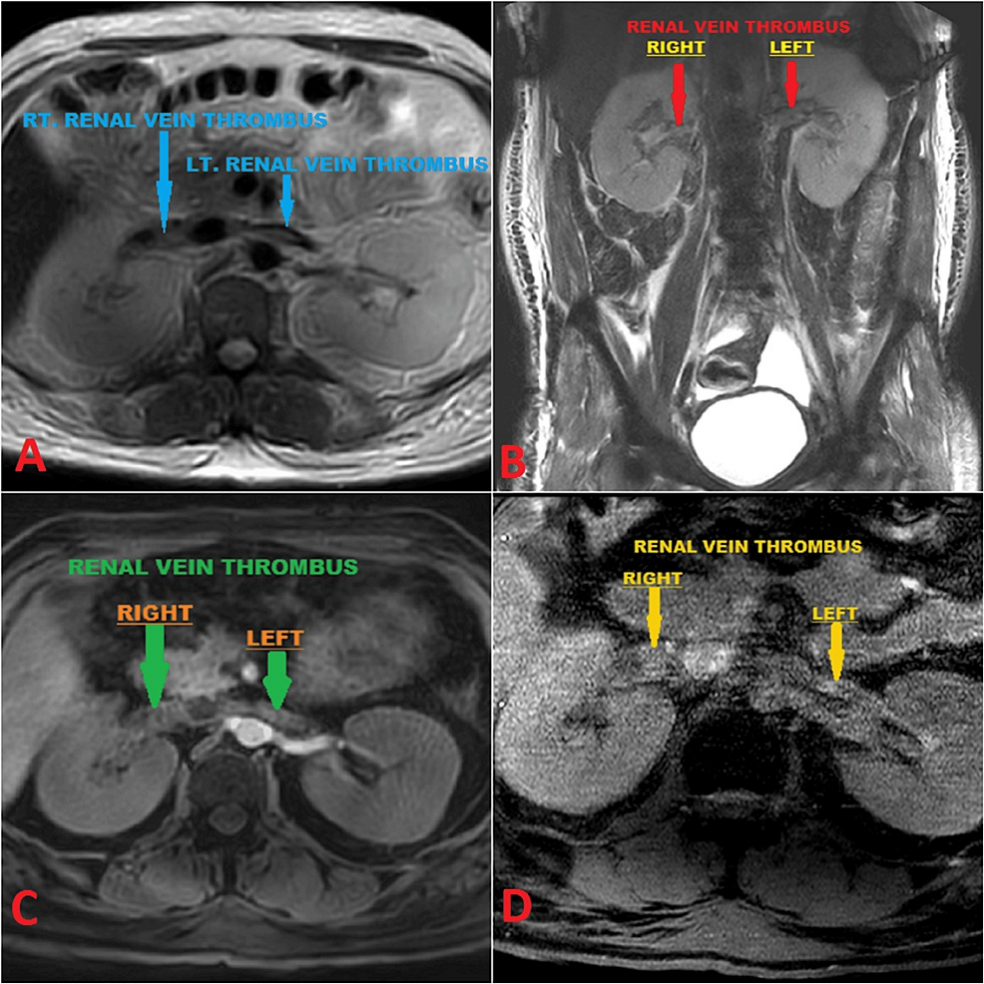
Bilateral renal vein thrombosis A: MR venography T2-weighted image depicting bilateral renal vein thrombus (blue arrows). B: MR venography T2-weighted image depicting bilateral renal vein thrombus (red arrows). C: 3D contrast-enhanced MR venography showing bilateral renal vein thrombus (green arrows). D: Partial thrombus in bilateral renal veins appearing as a filling defect in M2DI GS clear sequence (yellow arrows). MR: magnetic resonance
